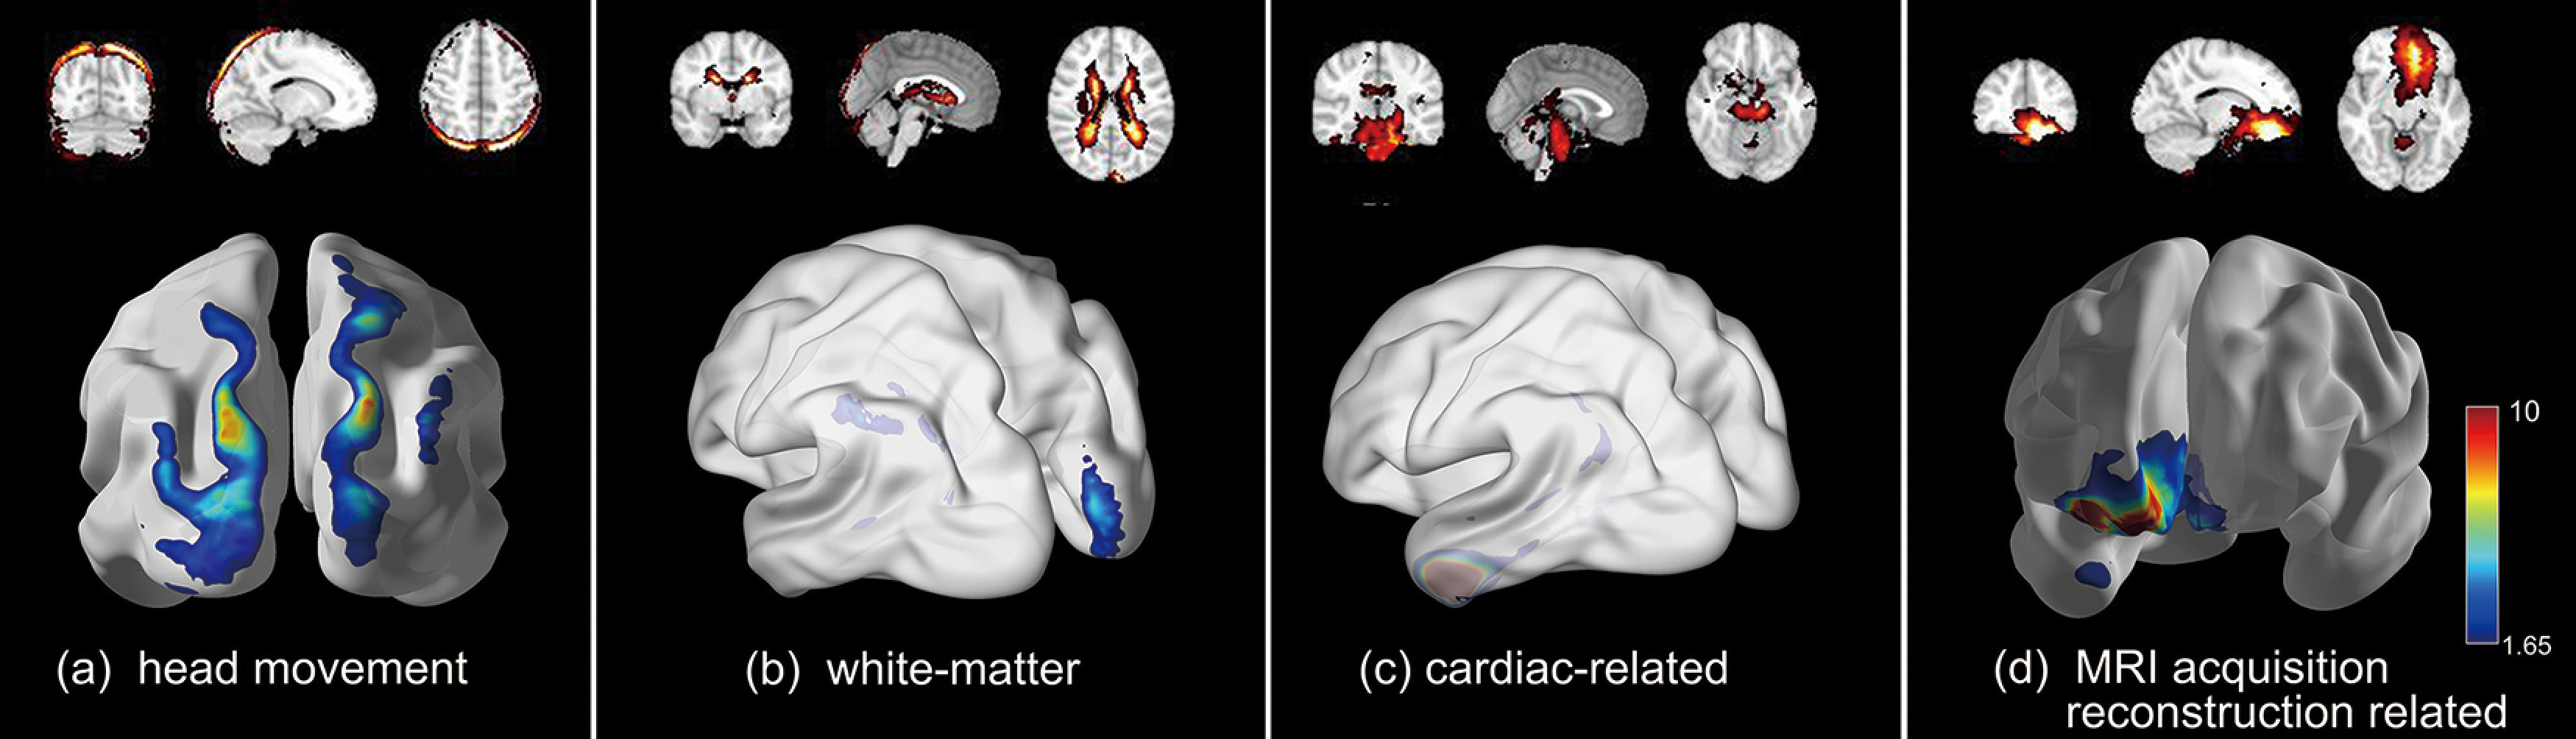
Artifact-related components detected by our framework, including (a) head movement, (*b*) white-matter, (*c*) cardiac-related, and (*d*) magnetic resonance imaging (MRI) acquisition/reconstruction related.
